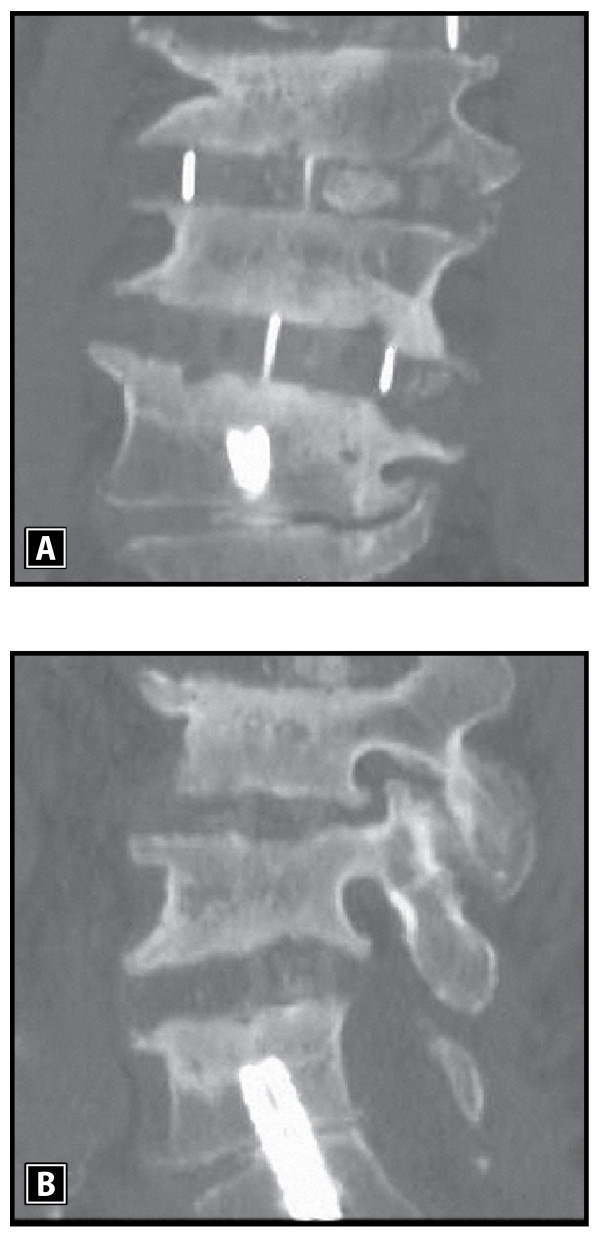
Coronal (A) and sagittal (B) computed tomography (CT) images demonstrating improved inter-vertebral L5-S1 disc space distraction and stabilization following minimally invasive AxiaLIF rod implantation with commensurate symptom resolution and functional improvement.
